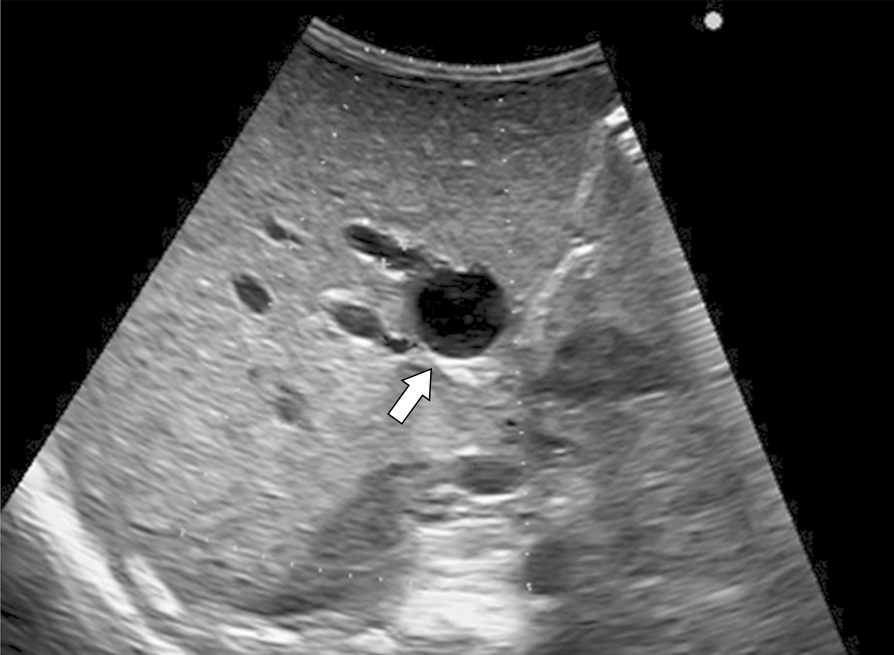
Ultrasonography at 37 days of age showed a biliary cyst at the porta hepatis (arrow)
